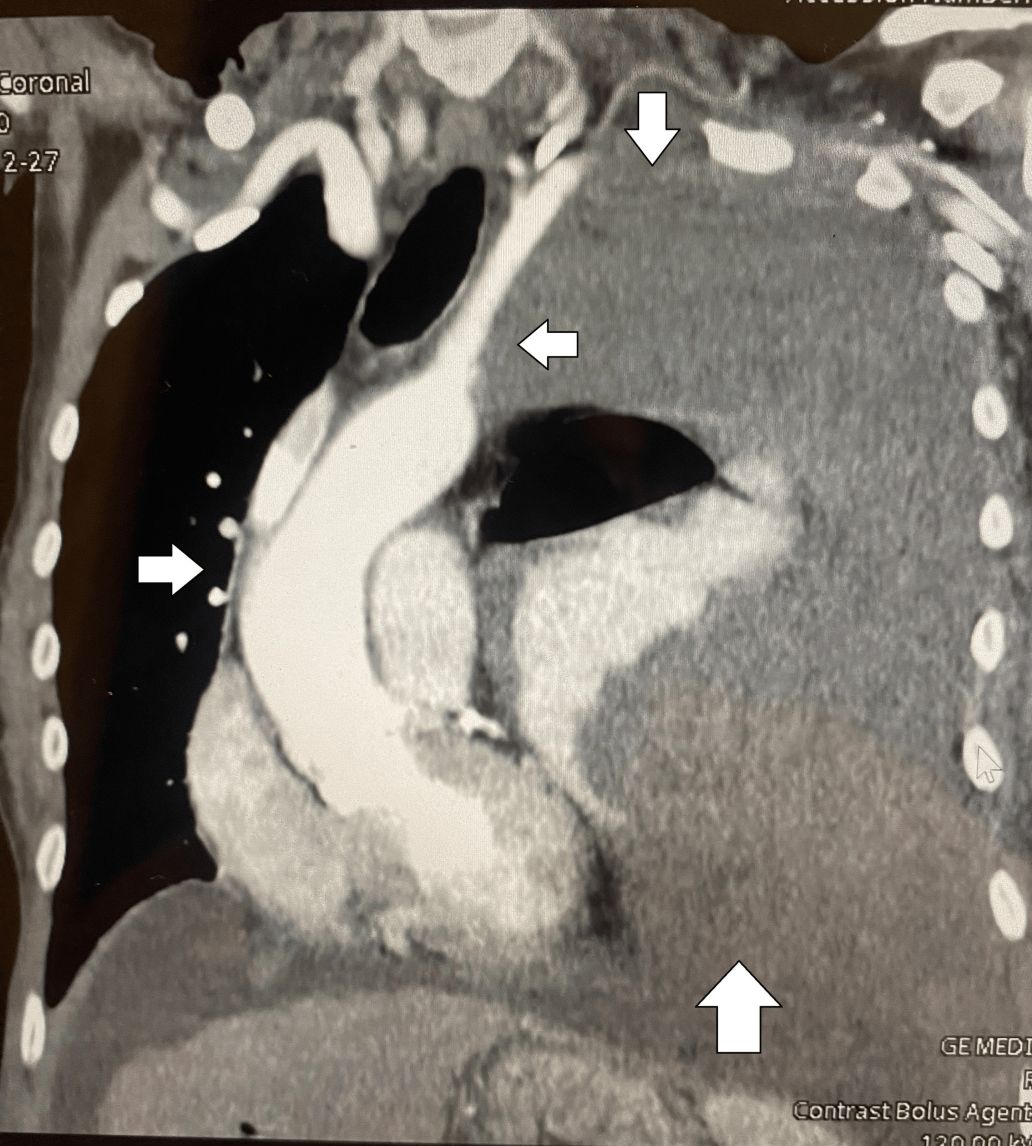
CT of the thorax with contrast sagittal view displaying tracheal deviation before thoracentesis.
